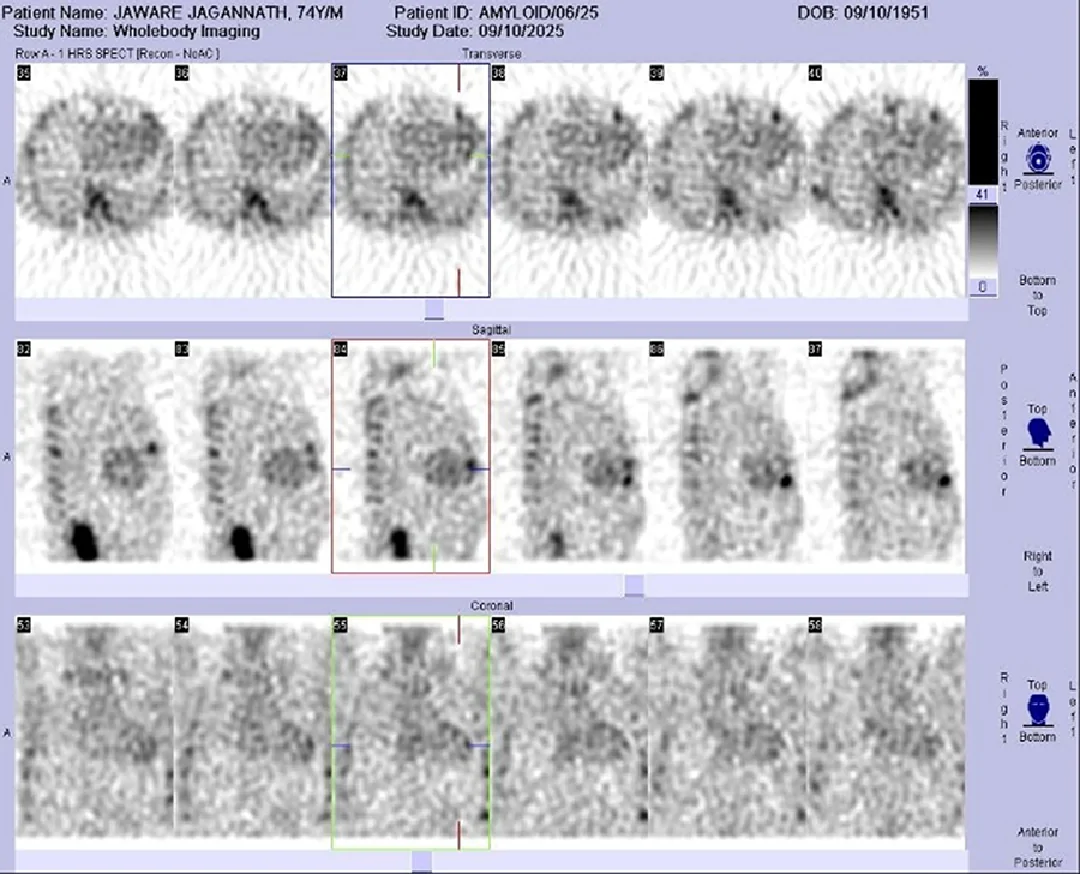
Single-photon emission computed tomography (SPECT) images acquired 1 h after 99mTc-pyrophosphate administration. Transverse, sagittal, and coronal reconstructions demonstrate diffuse radiotracer localization within the left ventricular myocardium, confirming true myocardial uptake and excluding blood-pool activity.
